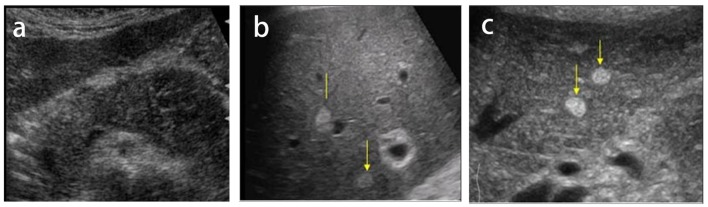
Ultrasound images (a, b, c) revealed edematous and heterogeneous pseudo-cystic changes on the body of pancreatic parenchyma (Department of Radiology in the University Clinical Center of Kosovo, Pristine).
